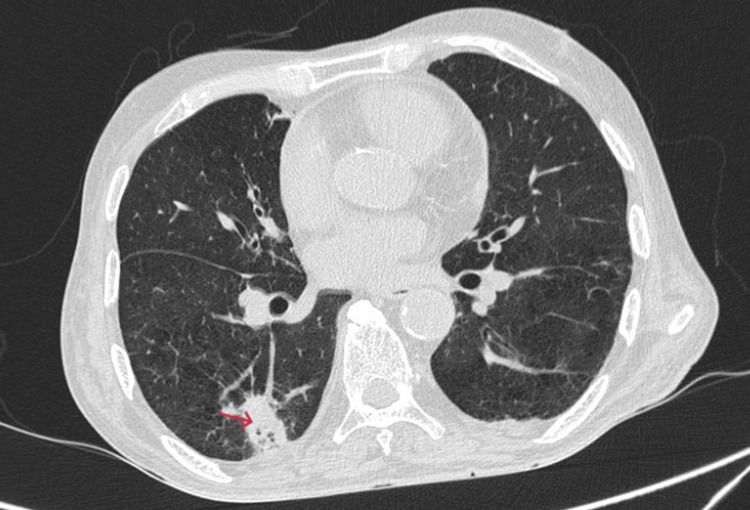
Axial CT lung window showing pulmonary infarct in the right lower lobe. CT, computed tomography
